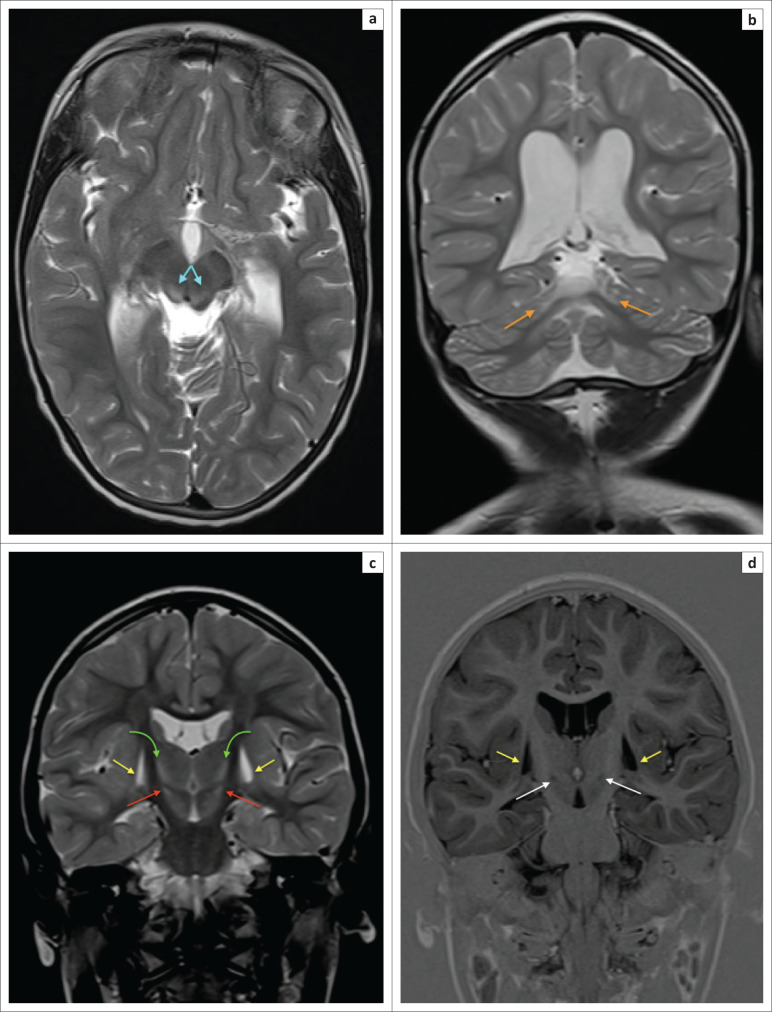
(a) and (b) are T2-weighted images in a 3-year-old female child with acute profound HIBI. (a) Dorsal midbrain tegmentum (cyan blue arrows) signal changes and (b) superior cerebellar (orange arrows) injury. (c) and (d) are coronal T2-weighted and IR sequences in a 7-year-old female child who presents with dyskinetic cerebral palsy after grade 2 hypoxic ischemic encephalopathy. (c) Hyperintensity at the subthalamic nucleus (red arrows) of the upper midbrain and (d) corresponding low signal intensity (white arrows) extending close to the periaqueductal gray (yellow arrows indicate putamen and green arrows indicate thalamus).
